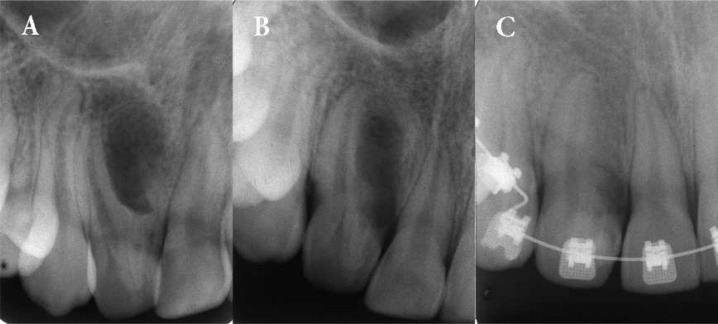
A)* Preoperative radiograph showing the tooth #7 with severe *type III* dens invaginatus with a large periradicular lesion; *B)* Ten days after surgical amputation of invaginated aberrant root; *C)* Four-year radiographic recall of involved tooth during an orthodontic treatment. Periapical radiograph showing complete healing of the periradicular lesion and the asymptomatic tooth responded a positive response to cold test
